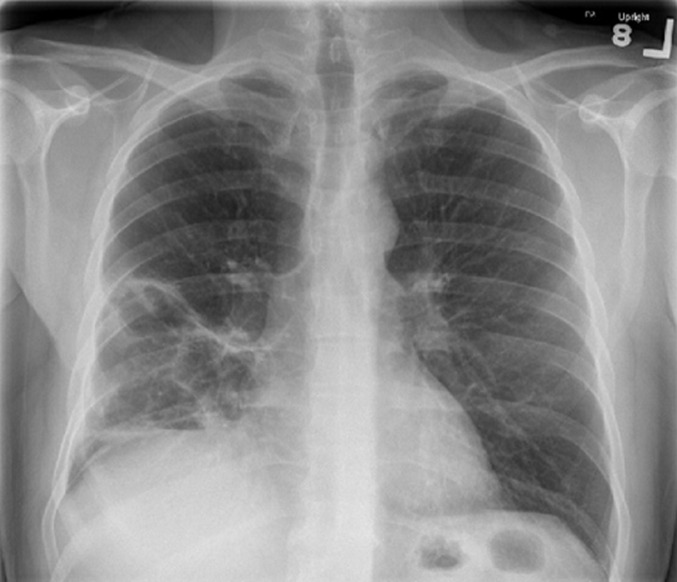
chest X-ray after drainage with a chest tube demonstrated significant improvement in lung aeration with residual pleural and basilar lung changes
